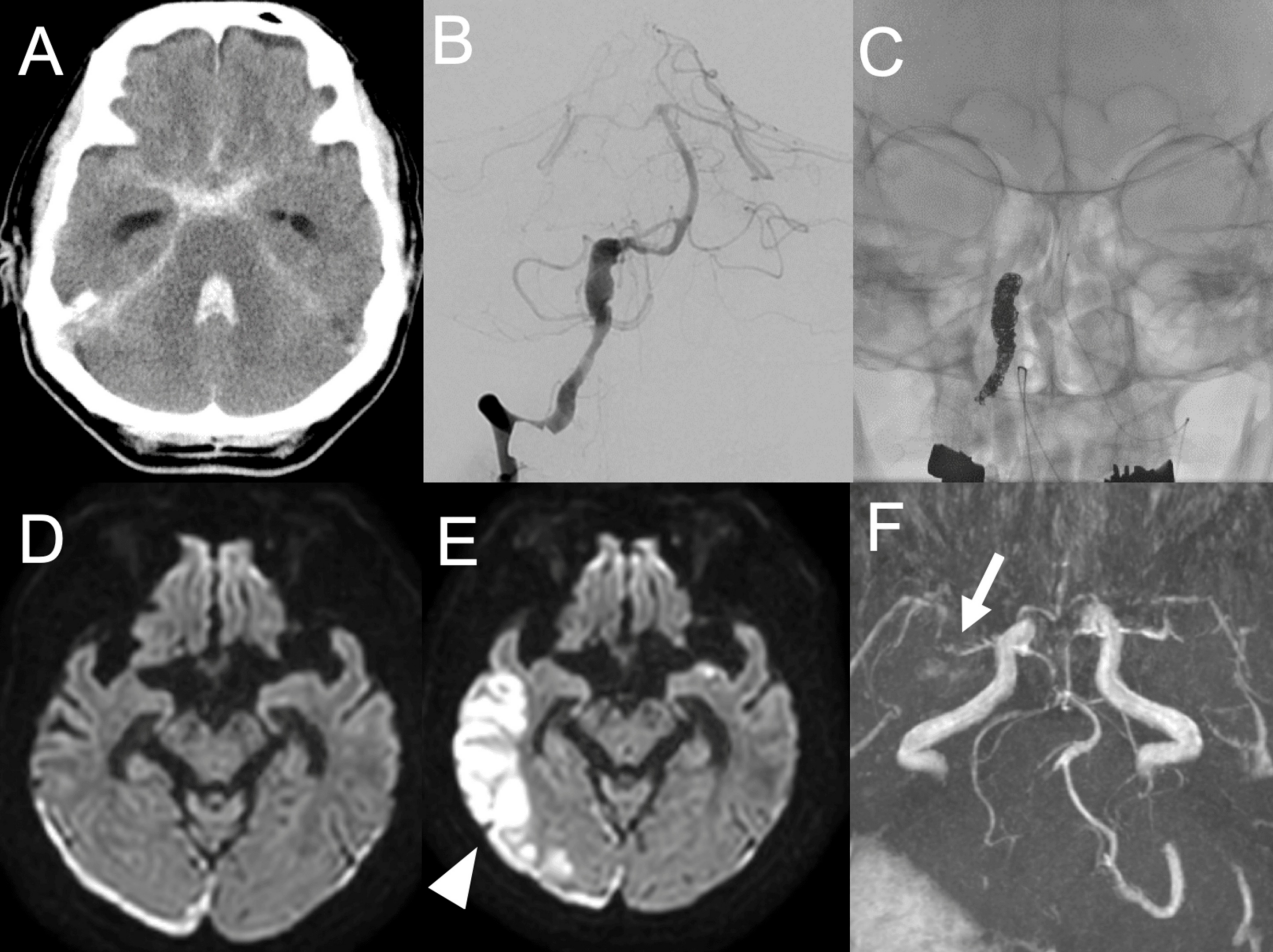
Neuroimaging findings (A) Head computed tomography at onset showing subarachnoid hemorrhage centered in the posterior cranial fossa with intraventricular hemorrhage. (B, C) Cerebral angiography of the right vertebral artery demonstrating irregular spindle-shaped dilatation, consistent with a ruptured dissecting vertebral artery aneurysm; parent artery occlusion was performed. (D) Diffusion-weighted head magnetic resonance imaging on postoperative day 14 showing no fresh infarction, with a high-signal area in the right temporo-occipital subdural region, consistent with subdural hematoma. (E) Diffusion-weighted magnetic resonance angiography of the head on postoperative day 16, after sepsis, demonstrating a fresh infarction in the right temporal lobe (white arrowhead). (F) Head magnetic resonance angiography on postoperative day 14 showing vasospasm in the right middle cerebral artery (white arrow).
